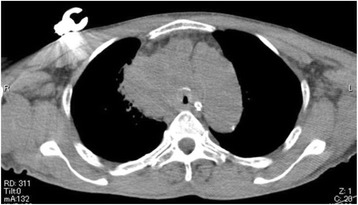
Chest CT after craniotomy. Chest CT showed that the maximum reduction in the tracheal cross-sectional area was estimated to be >90 %
